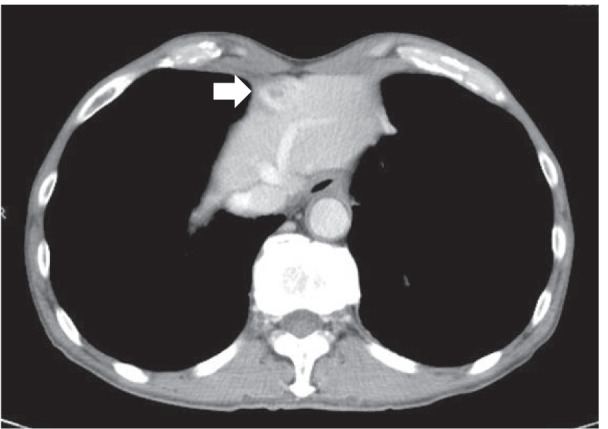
Contrast-enhanced computed tomography of the falciform ligament abscess at the time of the first admission. A 25-mm, enhanced mass containing small low-density areas was observed below the center of the diaphragm (white arrow)
